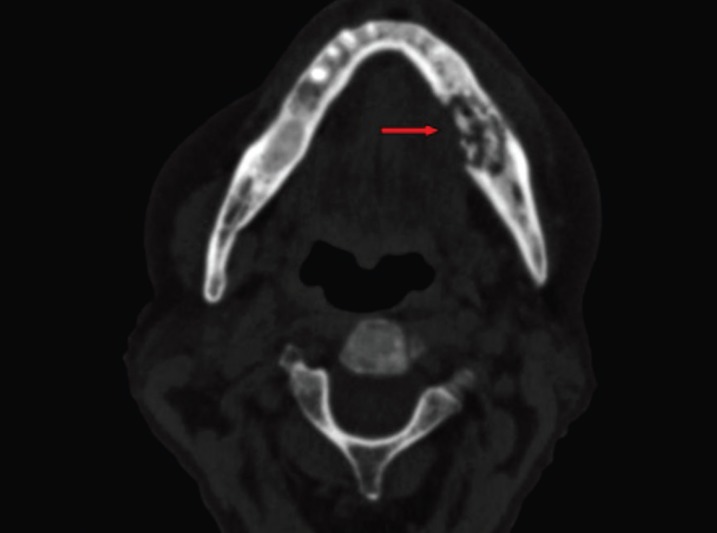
CT scan with osteosclerosis and bone sequestration areas, related to advanced stage BRONJ (red arrow).
